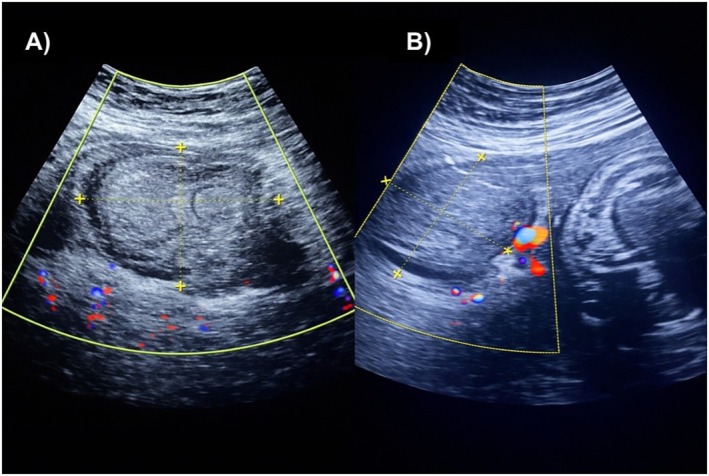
Transabdominal ultrasonography and color Doppler imaging demonstrating an enlarged right adnexal mass with heterogeneous echotexture and impaired vascular flow during late pregnancy. (A) Gray‐scale ultrasonography showing an enlarged, heterogeneous adnexal mass. (B) Color Doppler ultrasonography demonstrating no detectable intralesional vascular flow within the right ovary; adjacent vascular signals remain visible.
